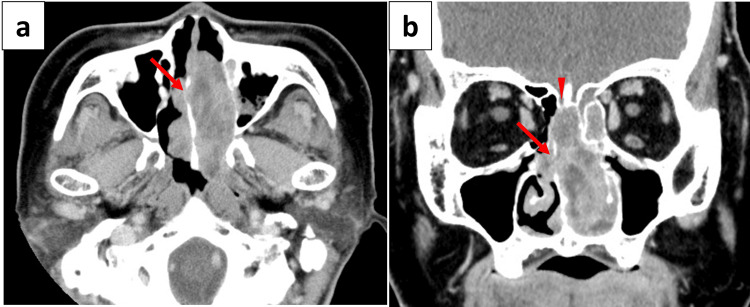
Preoperative sinonasal contrast CT findings. Axial (a) and coronal (b) sections showing the mass shadow with contrast effect centered on the nasal septum and extending into the left nasal cavity, left ethmoid sinus, and posterior nostrils, with no evidence of extension into the cranium, orbit, or hard palate, as interpreted by a board-certified radiologist at the time of initial evaluation. The arrows indicate the tumor. A retrospective review of the coronal image (b) reveals compression of the cribriform plate and subtle bone resorption, raising the possibility of involvement of the olfactory and ethmoid nerves (arrowhead).
